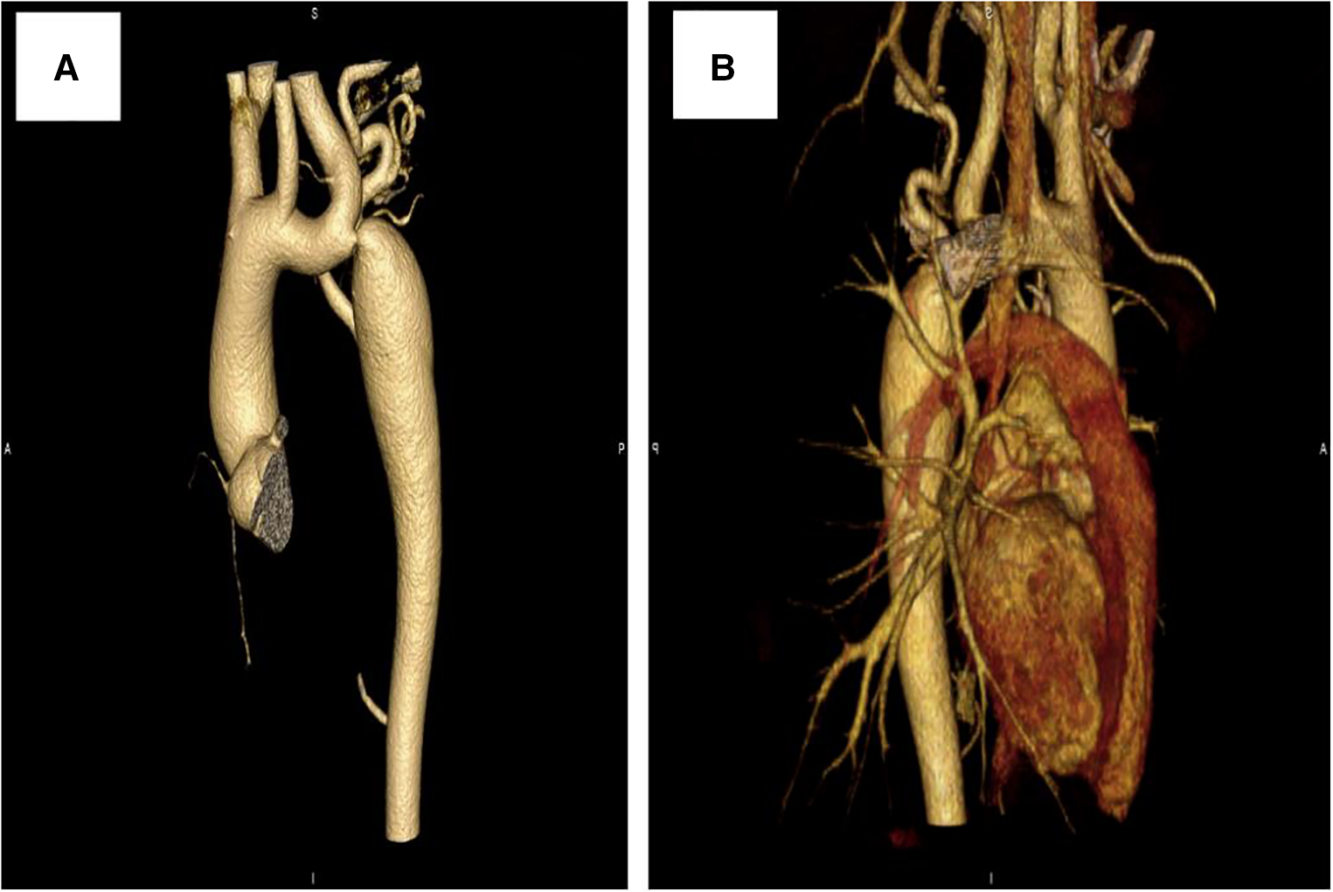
Computed tomography scan showing: (A) prior to stenting, (B) 3 years after stenting.
